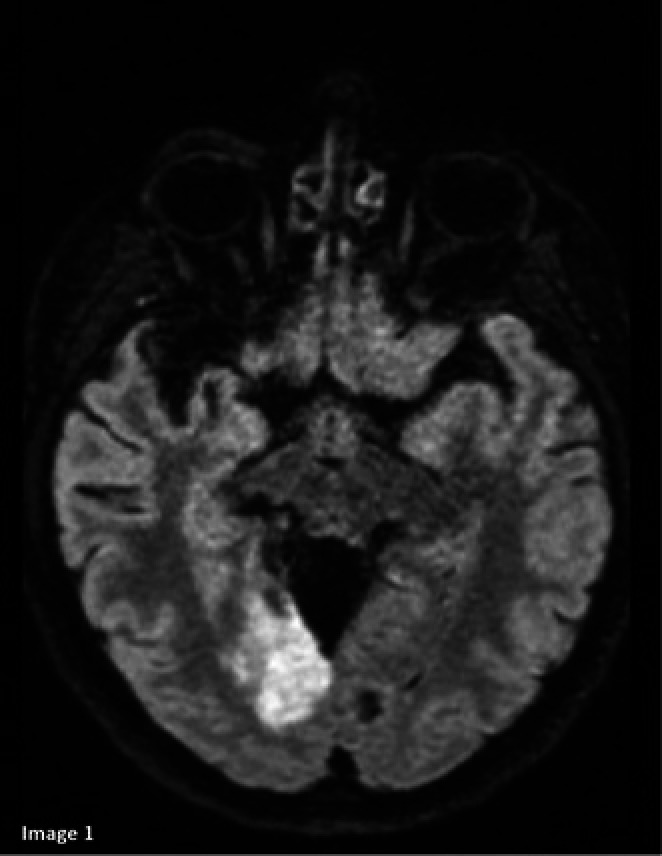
IMAGE 1 Axial FLAIR MRI showing hyperintense signal in the right occipital lobe.
